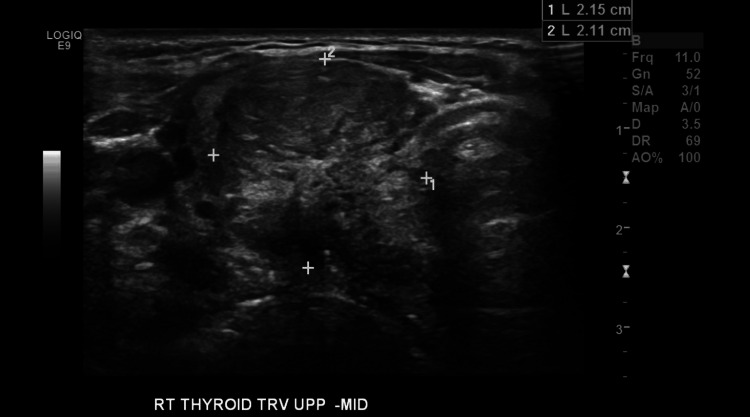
Transverse ultrasound of the right thyroid, upper-middle lobe. The ultrasound shows a 2.15 x 2.11 cm nodule. RT THYROID: right thyroid; TRV: transverse; UPP-MID: upper-middle
